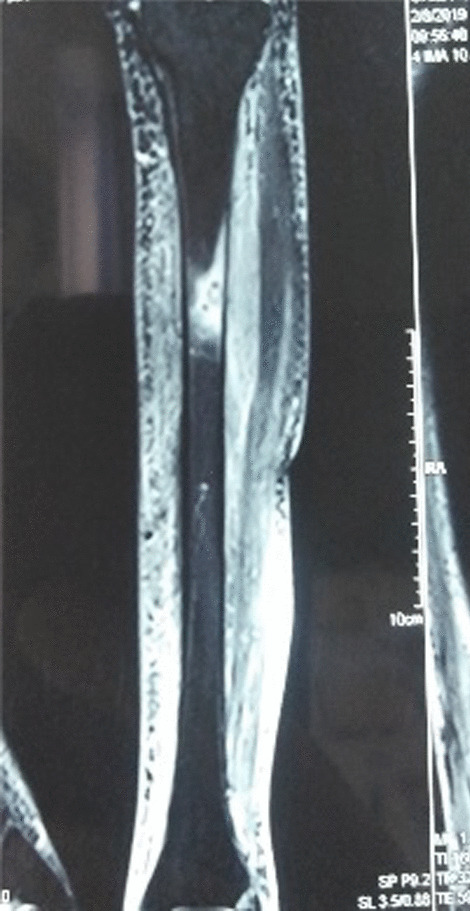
MRI axial view of the affected tibia, STIR sequence showing a high-signal, osteolytic-like lesion of the diaphysis
